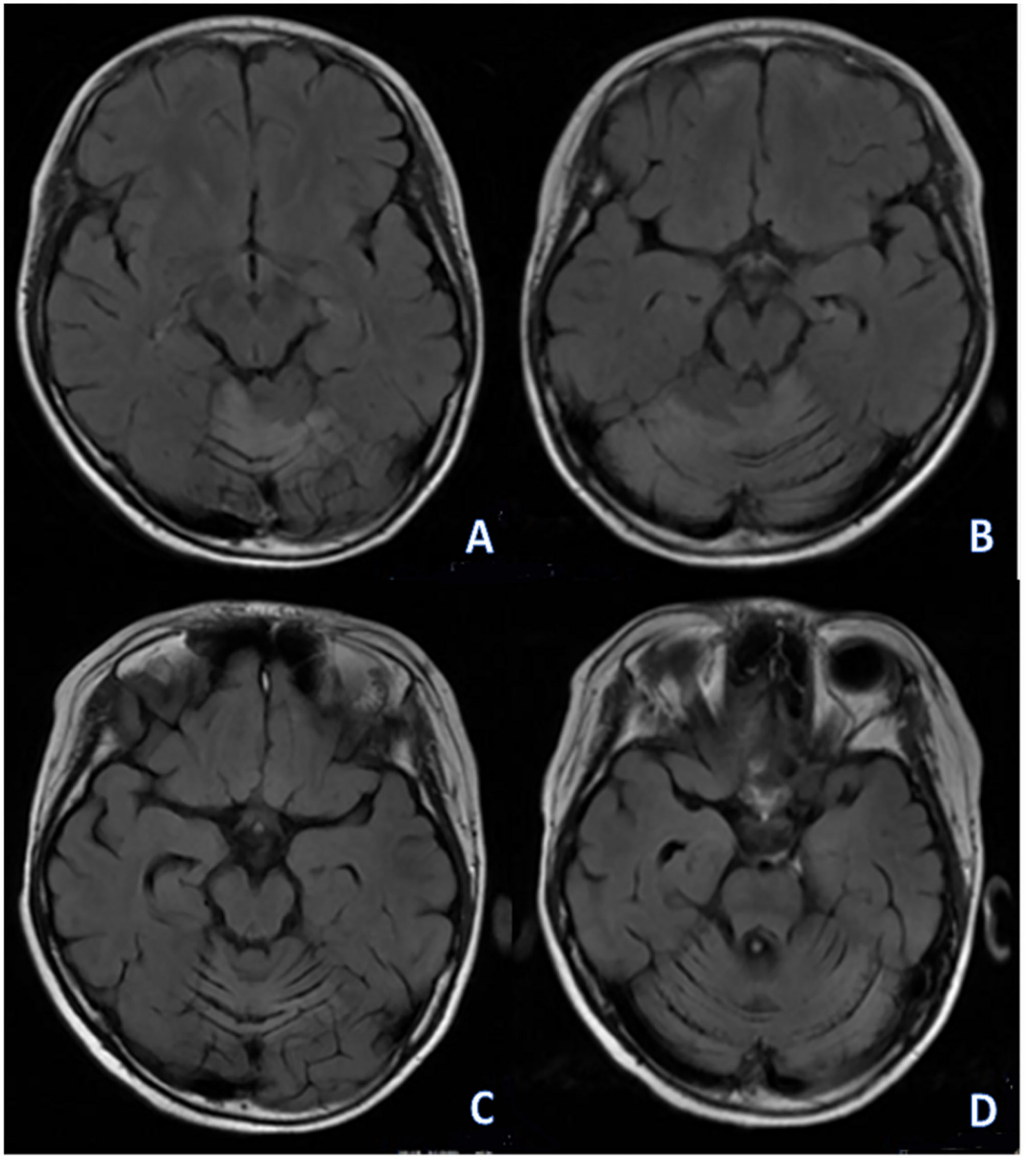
(A,B) initial MRI: right cerebellar atrophy and multiple lesions in bilateral cerebellum and vermis. (C,D) follow-up MRI showed bilateral cerebellum and vermis atrophy, sulcus of left cerebellum was wider.
